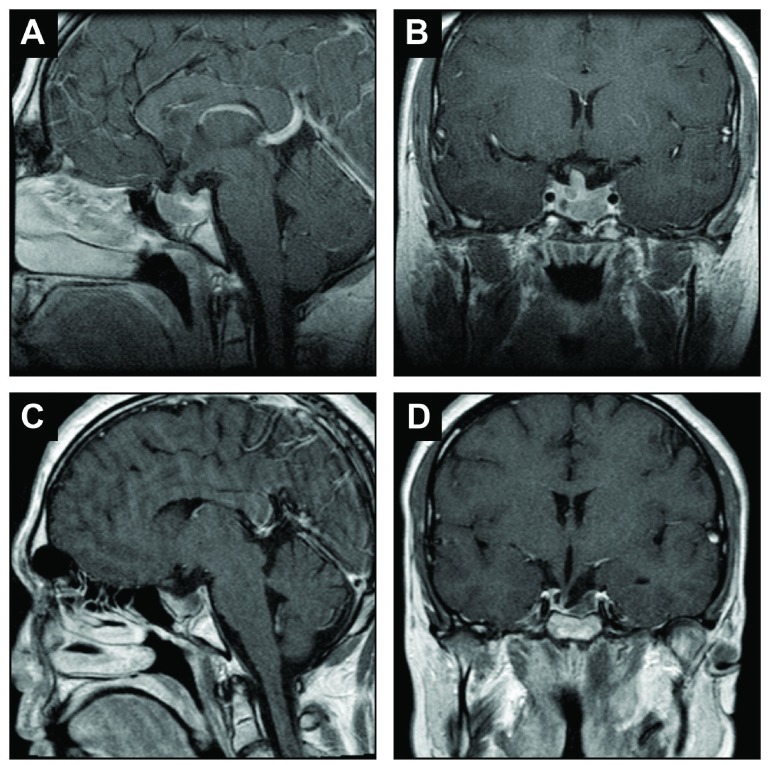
Magnetic resonance imaging: pre-treatment (
